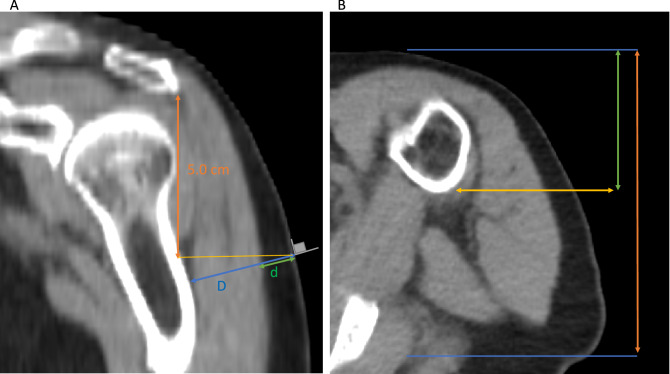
(A) Coronal CT image of a 77-year-old man with history of head and neck squamous cell carcinoma, weight of 74 kg, height of 1.70 m, demonstrating the deltoid subcutaneous fat pad (DSFP) measurement technique. Orange arrow identifies the region exactly 5.0 cm inferior to the acromion. Yellow line is perpendicular to the orange line and identifies the estimated injection site. 90° angle at the estimated injection site in gray was used to identify the trajectory of the needle. Green arrow identifies the distance from the skin surface to the deltoid muscle measured perpendicular to the skin in the coronal plane (note arrow is jittered to show blue arrow along the same path) (DSFP = d = 11.2 mm). Blue arrow identifies the distance from the skin surface to the humerus measured perpendicular to the skin in the coronal plane (D = 40.3 mm). (B) Axial CT image of the same man demonstrating identification of the correct coronal plane to measure the DSFP. Blue lines identify the anterior and posterior borders of the shoulder 5.0 cm inferior to the acromion. The orange arrow measures the distance between the anterior and posterior borders of the shoulder. The green arrow measures the half-way distance between the anterior and posterior borders of the shoulder. The yellow arrow was used to identify the coronal plane to take measurements of the deltoid subcutaneous fat pad.
